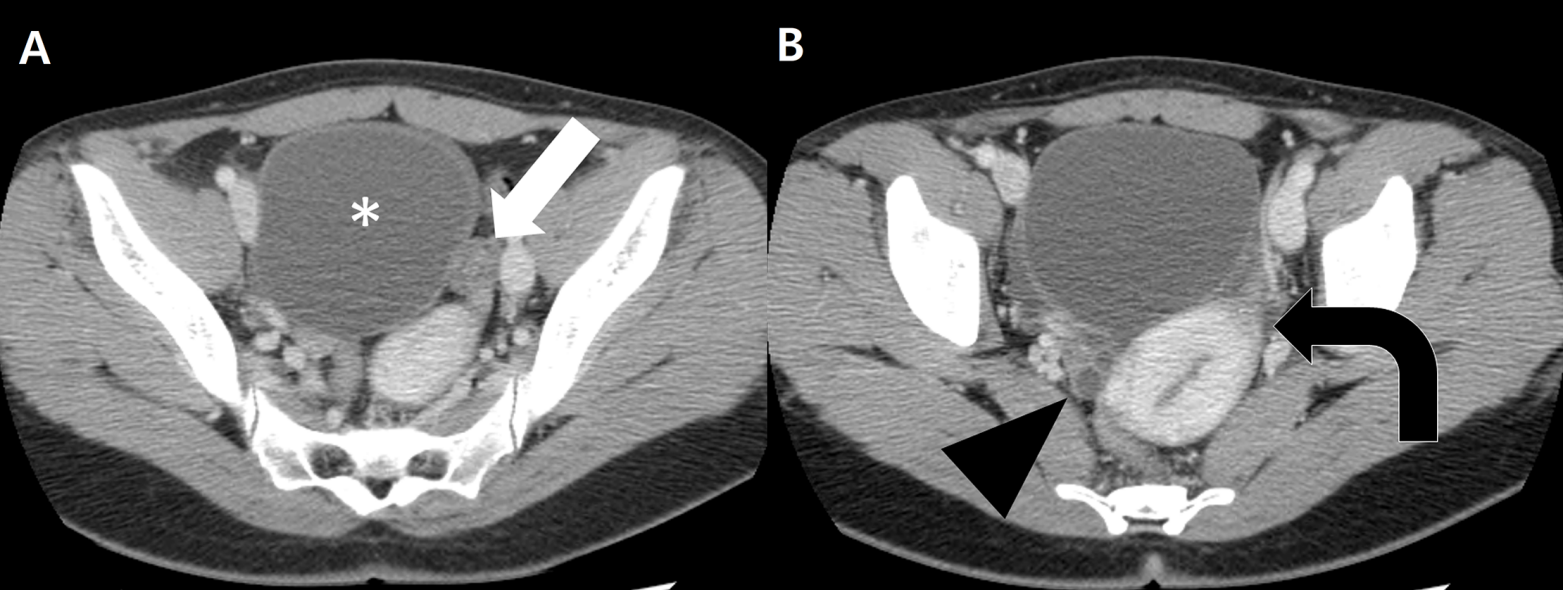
A 23-year-old woman with torsed left ovarian mucinous cystadenoma. A, Axial contrast-enhanced CT scan shows a thickened pedicle (arrow) between the left uterine cornu and a pelvic mass (asterisk). B, CT scan obtained caudal to image A shows uterine deviation (curved arrow) toward the thickened pedicle. Note that the right ovary (arrowhead) is seen separately.
